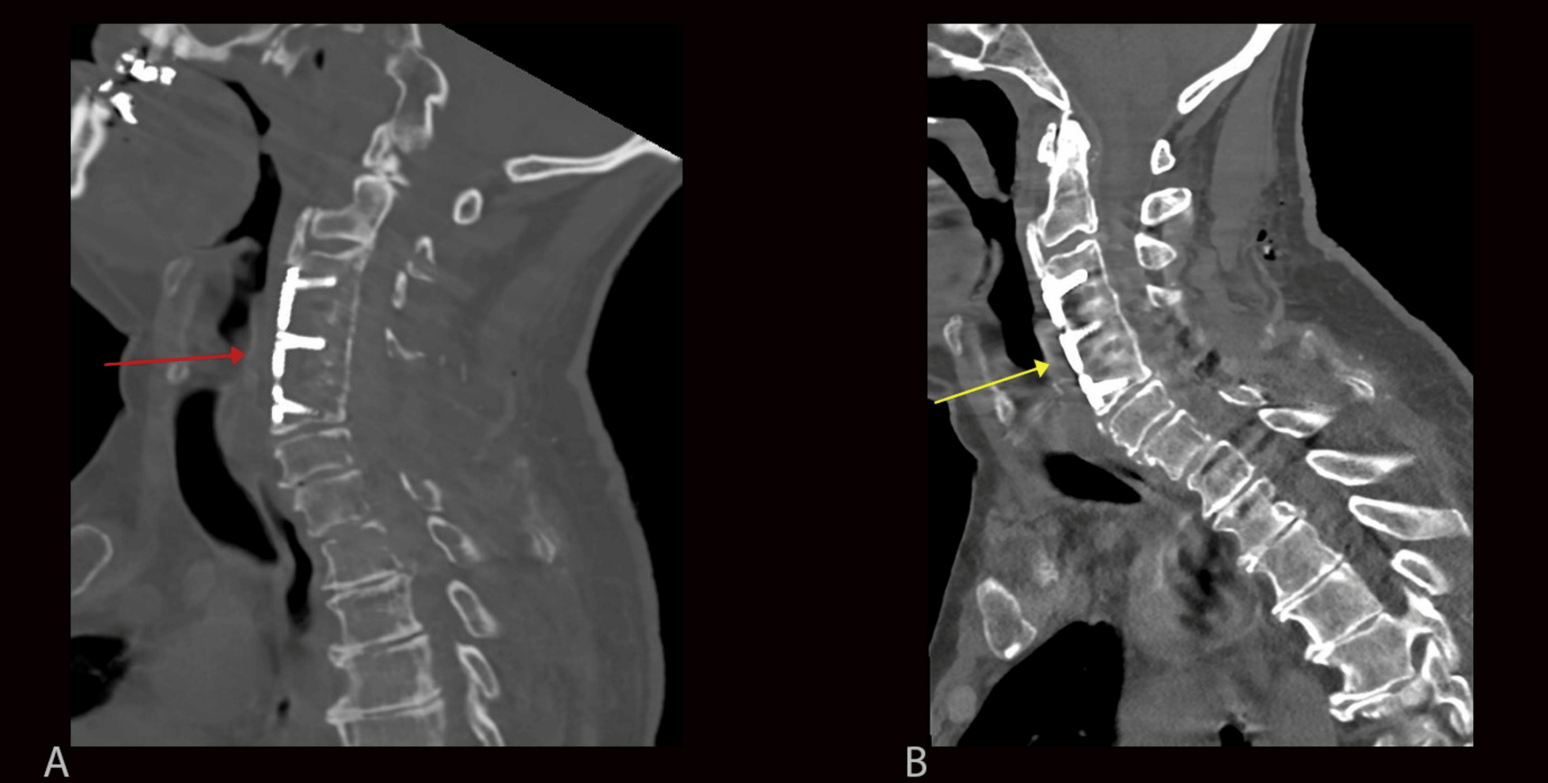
Sagittal images of the cervical spine with iMAR post-processing (A) and without iMAR post-processing (B) Figure 2A shows an iMAR post-processed image with lucencies surrounding the anterior cervical spine fixation hardware (red arrow). These lucencies do not persist on sagittal CT images without iMAR post-processing (yellow arrow, Figure 2B) but rather demonstrate normal trabecular bone with fairly minimal overlying streak artifact. These findings suggest the absence of hardware loosening and are consistent with iMAR-generated artifacts. iMAR: iterative metal artifact reduction
